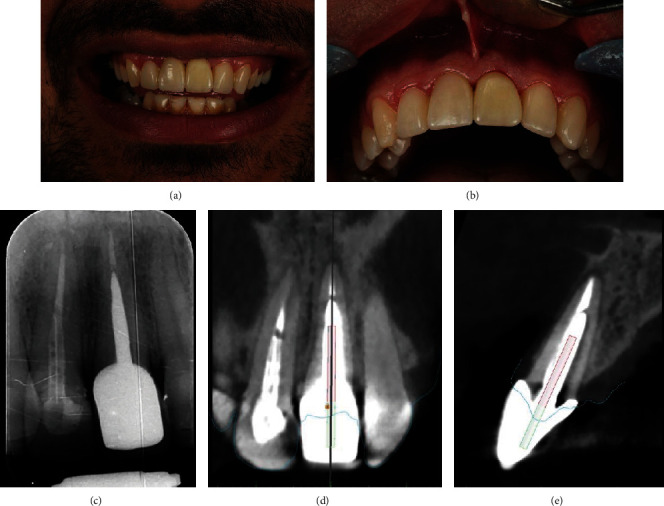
(a) Postoperative clinical view of a permanent restoration. (b) Clinical view of 6 months postoperatively. (c) Radiograph view of 6 months postoperatively showing periapical healing. (d, e) Coronal and sagittal view of postoperative CBCT showing the dimensional reduction of the periapical lesion. The superimposed preplanned pathway is located in the center of the canal.
